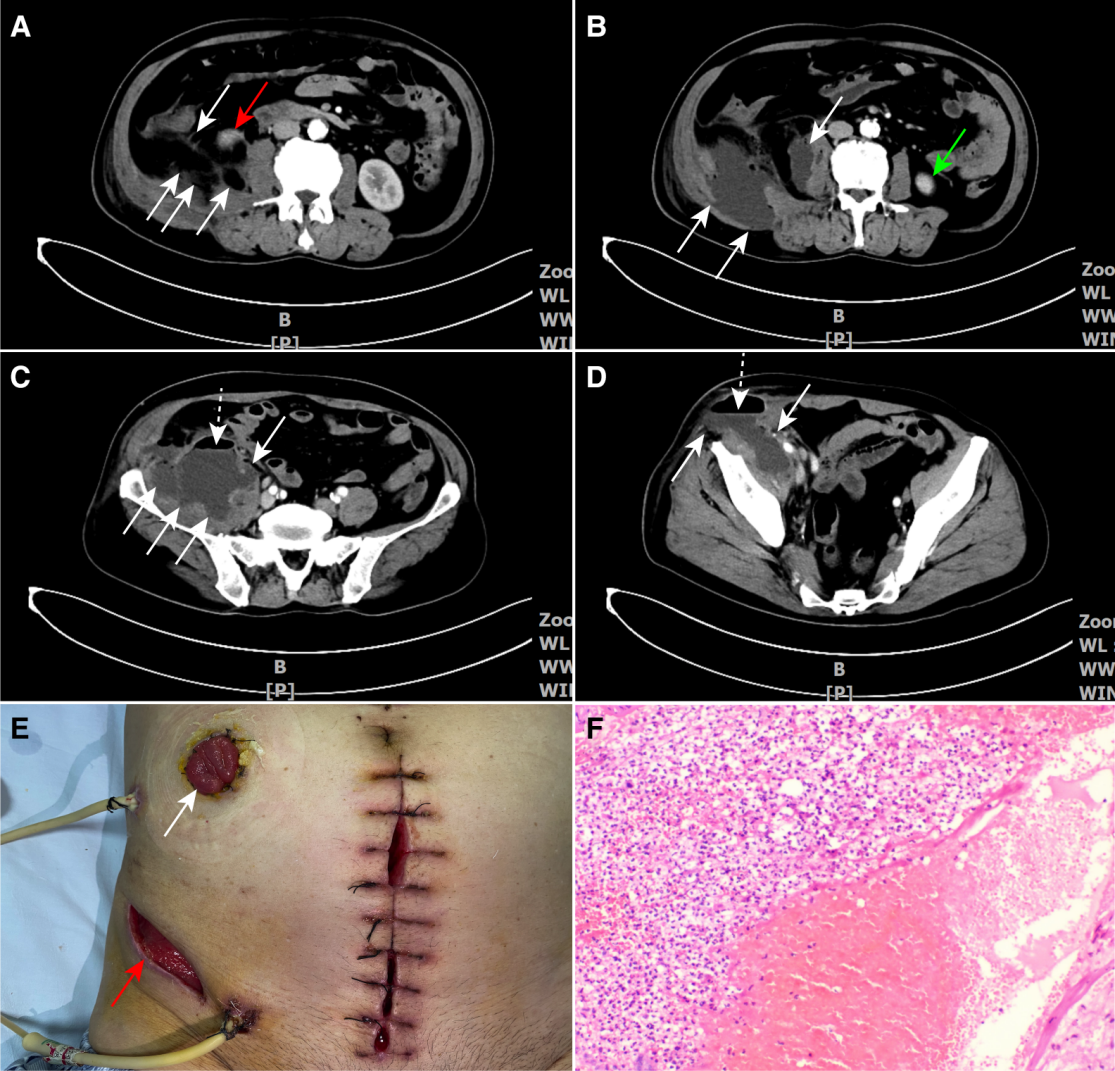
(A) The white arrows depict the gas-filled sections of the iliopsoas abscess. The red arrow points to the inferior pole of the right kidney. (B–D) CT scans reveal the extent of the iliopsoas abscess, with the white arrows outlining the abscess borders, the green arrow pointing to the inferior pole of the left kidney, and the white dotted arrows indicating the gas–fluid levels in the upper part of the abscess. (E) The postsurgery image displays the extra-abdominal area. The white arrow shows the stoma of the terminal ileum, and the red arrow highlights the incision made on the lateral side of the groin. (F) The postoperative pathology report confirms gangrenous appendicitis. CT, computed tomography.
